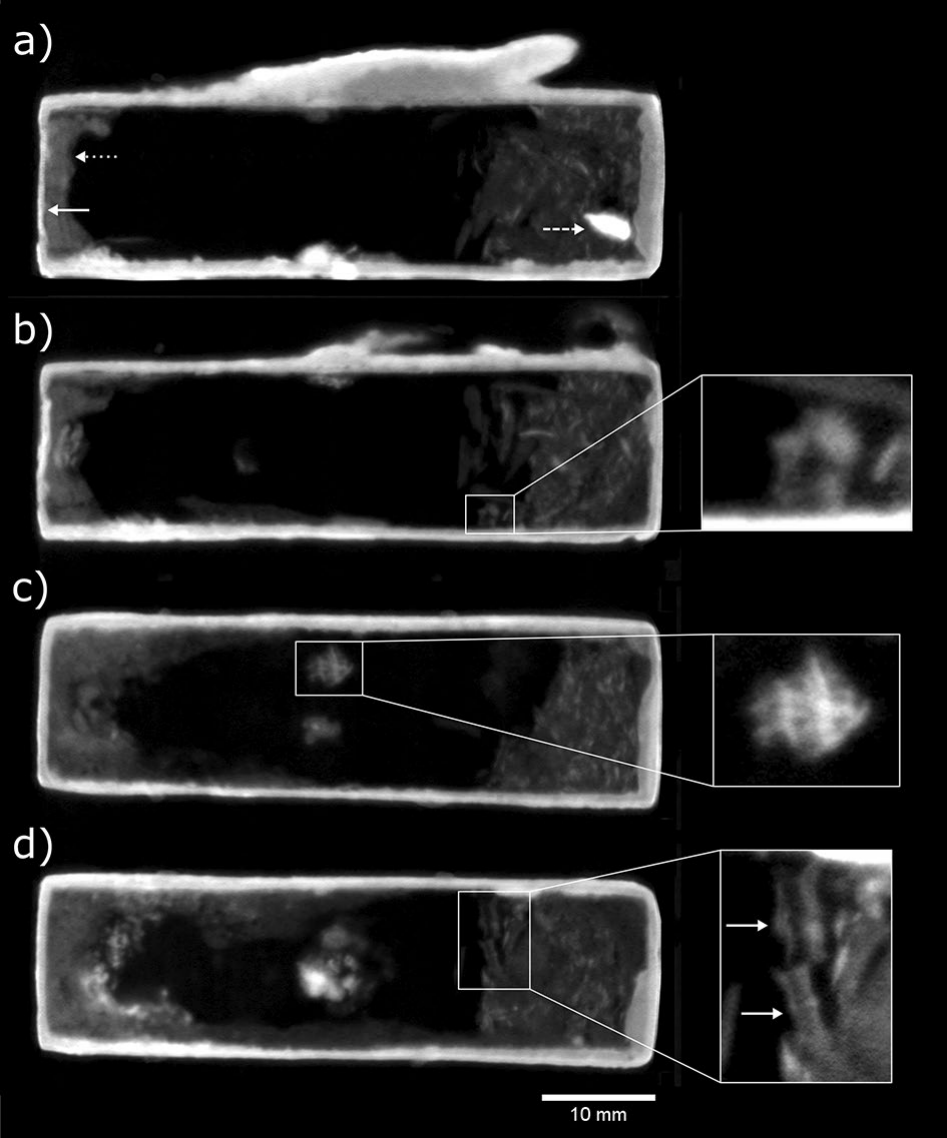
EA49146 multiplanar neutron CT slices, averaged over 0.44 mm slice thickness: (a) side-view slice centred 7.0 mm from right side of box (solid arrow: metal plate covering plug, dotted arrow: plug, dashed arrow: loose chaplet); (b) side-view slice centred 11.7 mm from right side of box (inset: vertebra, transverse cross-section); (c) top-view slice centred 13.8 mm from base of box (inset: textile fragment); (d) top-view slice centred 2.1 mm from base of box (inset: vertebrae, longitudinal cross-section, indicated by solid arrows).
